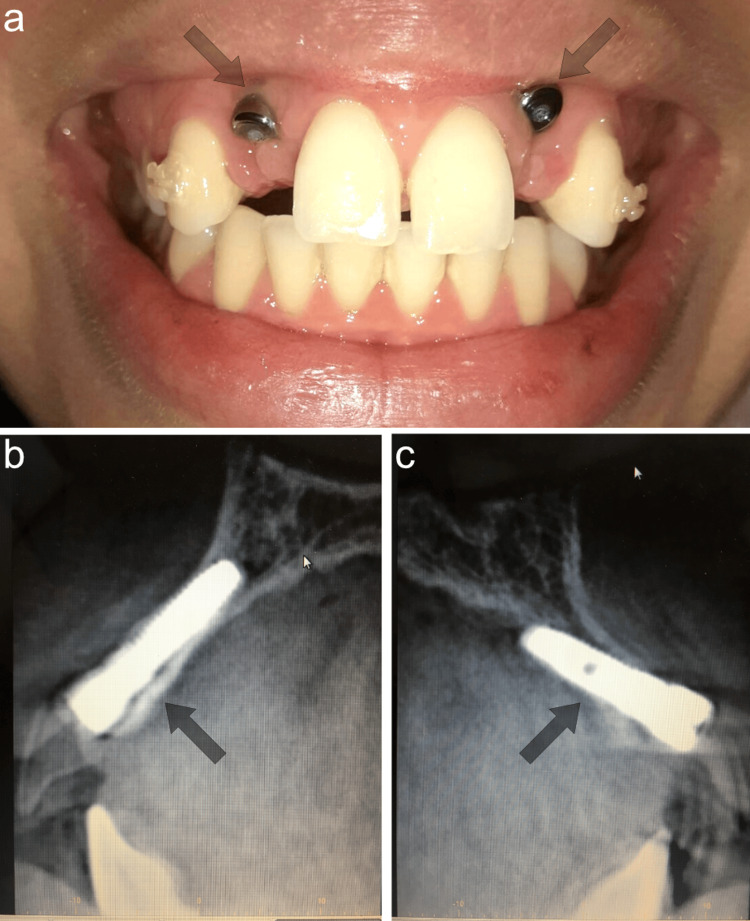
Baseline intraoral clinical view (a) and corresponding radiographic image showing implants located at sites 1.2 (b) and 2.2 (c) at the initial presentation.
